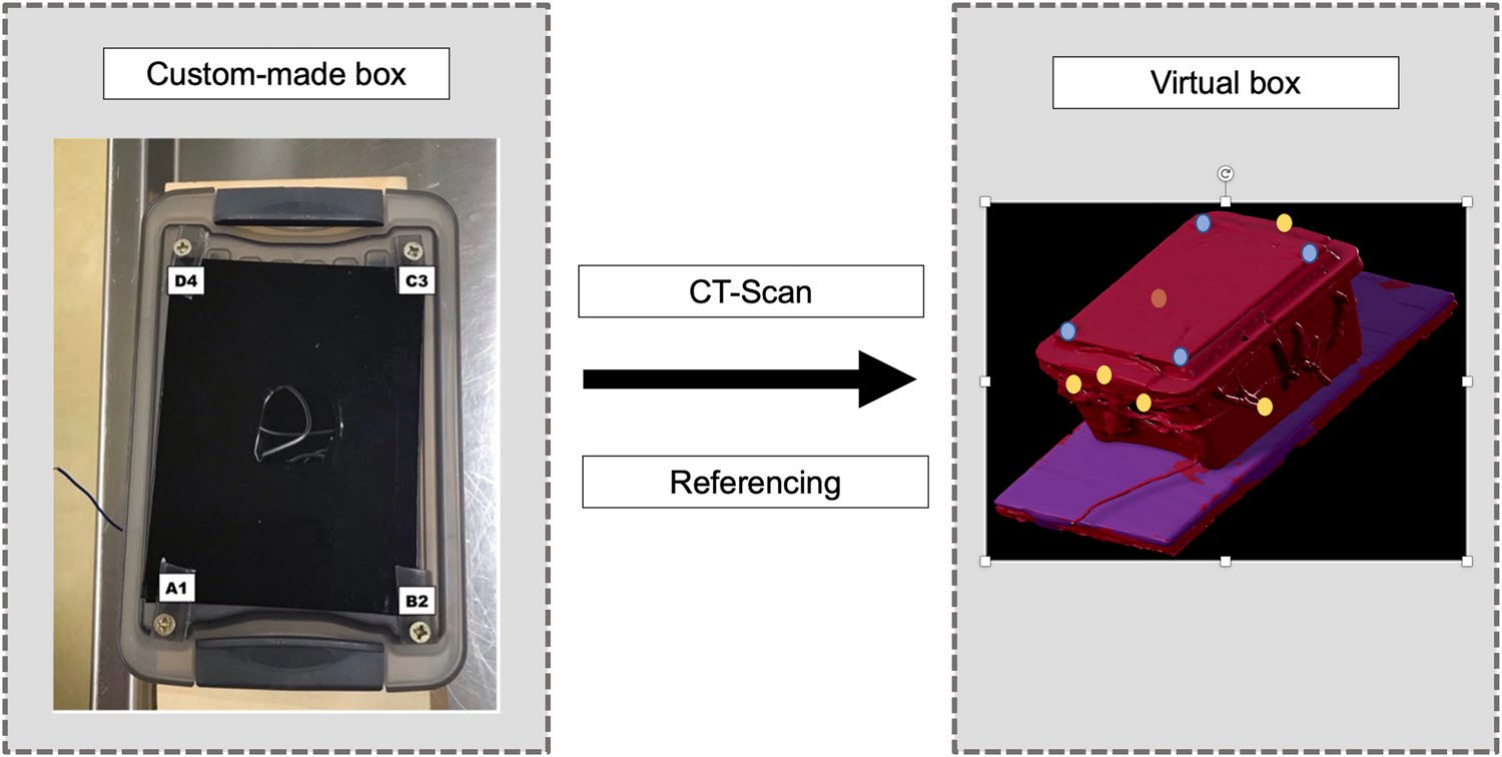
A plastic box was custom-made by fixing four metallic skews on the top of the box representing the four target points A1, B2, C3, D4. The box was scanned and referenced. Virtual objects were defined from the CT for the purpose of the neuronavigation. The blue dots represent the virtual screws (targets). The yellow dots represent the pre-defined points for the reference process of the box
